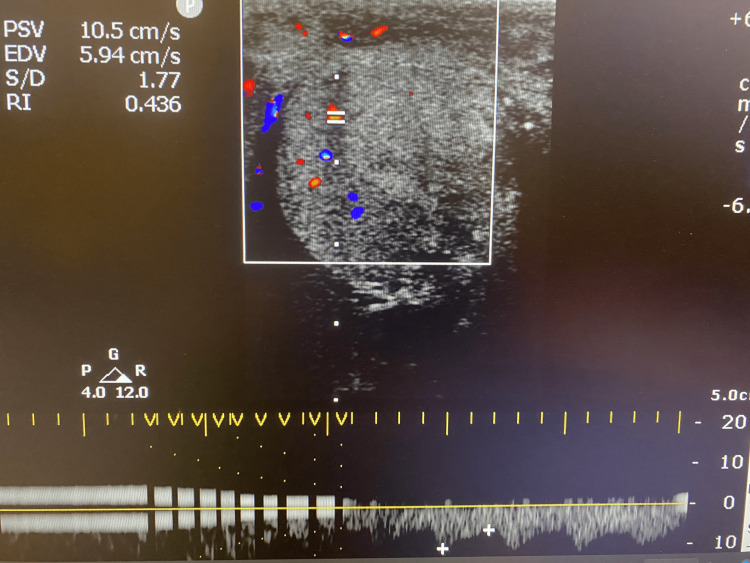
Doppler ultrasound of the left testis revealing preservation of arterial blood flow The figure is presented with the patient’s informed consent.
